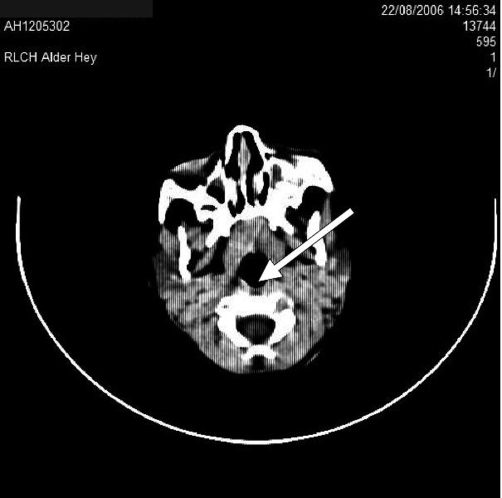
Axial computed tomography scan of the head and neck taken approximately 4 weeks after treatment initiation showing complete resolution of retropharyngeal abscess.
